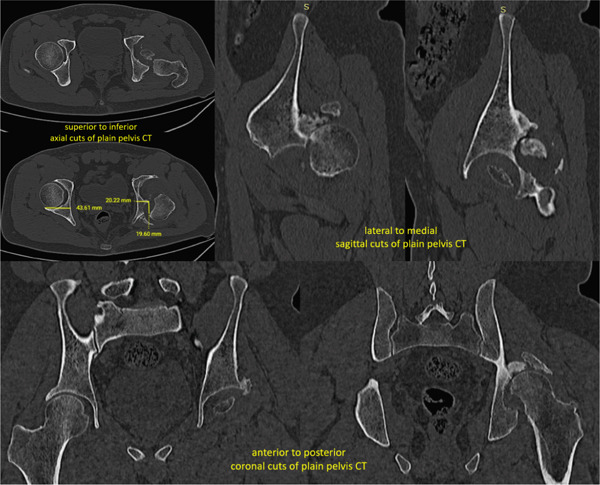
Case 2 preoperative computed tomography.
